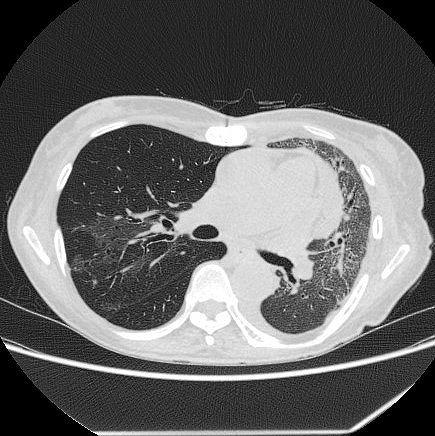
Chest computed tomography before discharge showed that consolidation and ground-glass opacity were improved.
